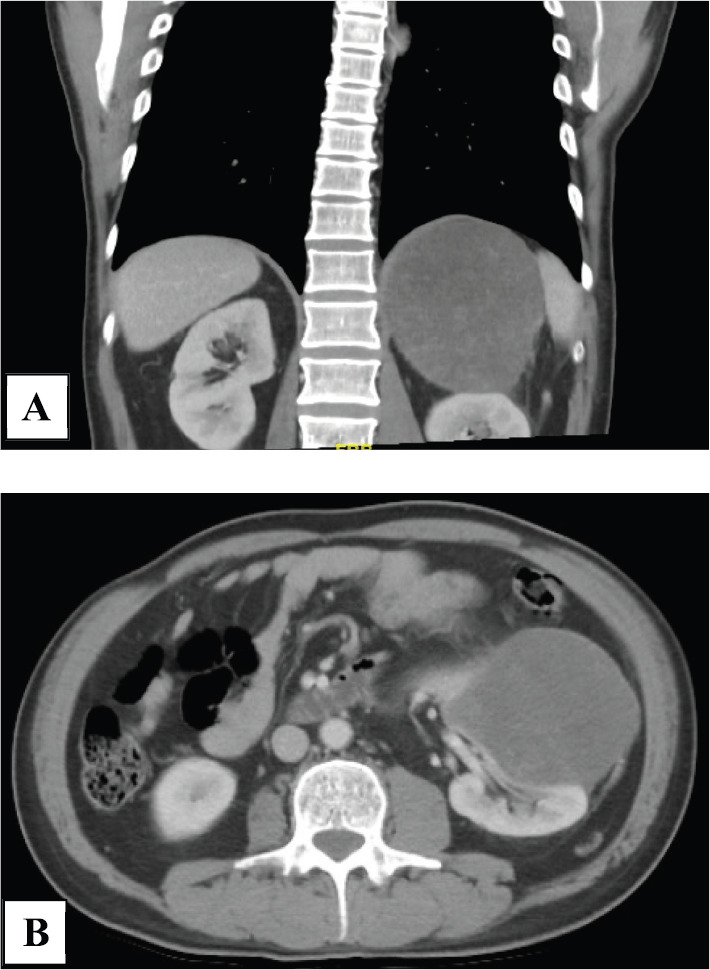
Computed tomography scan. (a): Abdominal CT scan showing a neoplastic lesion in the left kidney of heterogeneous density and (b): with alteration of the adjacent fat planes in its caudal pole.
